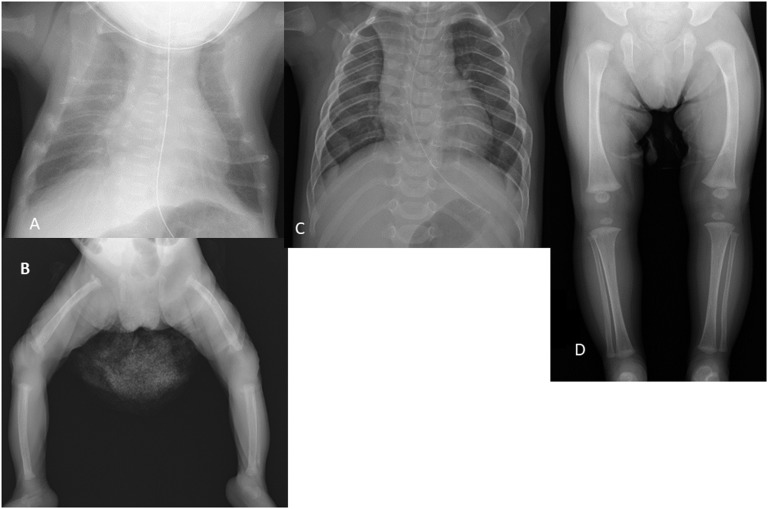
X-ray findings of the patient. (A, B) Neonate. Osteopenia of the skeleton is observed. The ribs are thin and wavy, the thorax is bell shaped and narrow, and the cortex of the rims is coarse, thin, and markedly demineralized. The femora are mildly bowed, and metaphyseal constriction resulting from fracture is observed (arrow). (C, D) Six months of age. The skeletal lesions almost completely resolved.
